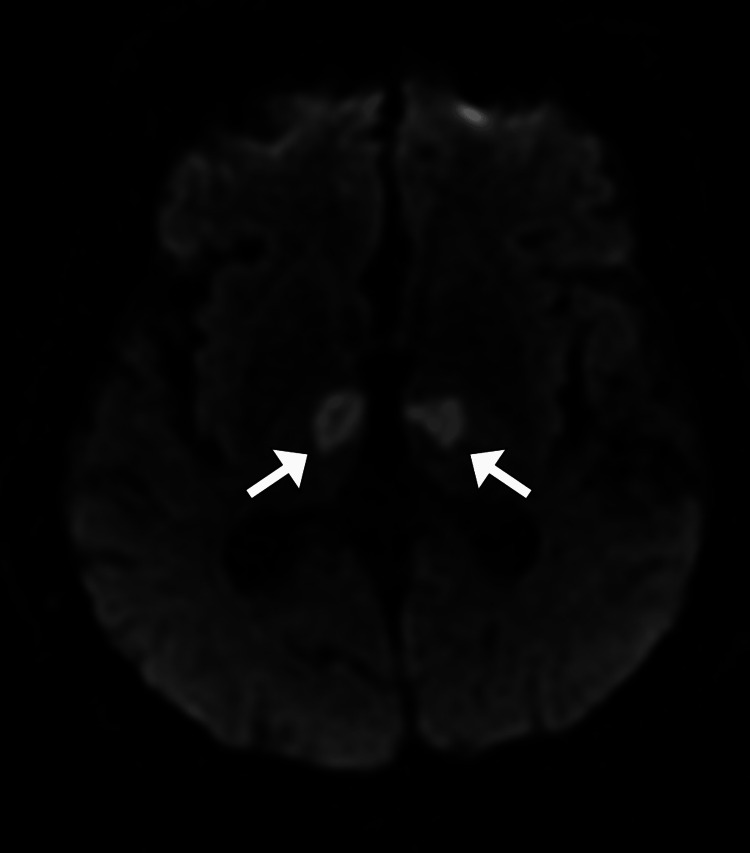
Axial DWI image of brain MRI shows areas of restricted diffusion (arrows) in paramedian thalami. DWI: diffusion-weighted imaging; MRI: magnetic resonance imaging
